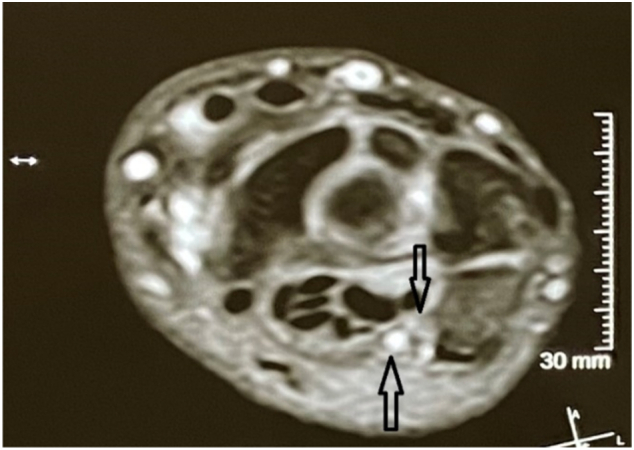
MRI show Guyon's canal with a mass inside.
